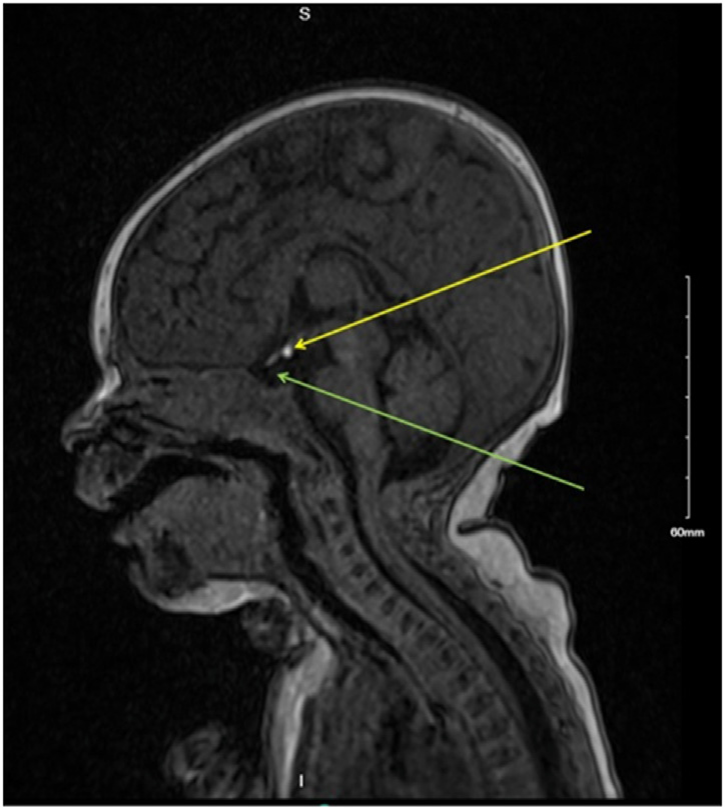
Magnetic resonance imaging of the brain (sagittal view); *yellow arrow* shows posterior pituitary gland; *green arrow* shows empty sella turcica.
